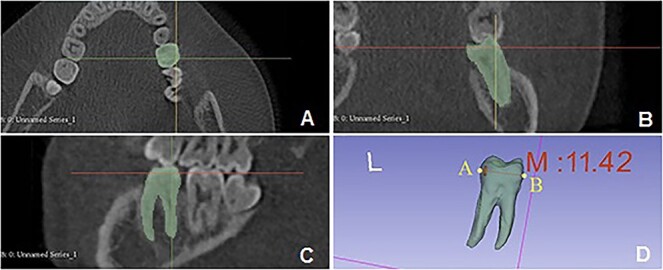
The measurement values in the 3D Slicer software. Make the cone-beamcomputedtomography (CBCT) image segmentation of mandibular first molars as an example: the green part shows the selected area of the teeth in vertical direction; (A) coronal direction (B), and sagittal direction (C). (D) The green model is the segmented mandibular first molars from CBCT image by 3D Slicer, and then to calculate the volume; the distance between the A and B points is the mesiodistal diameter (mean = 11.42 mm).
